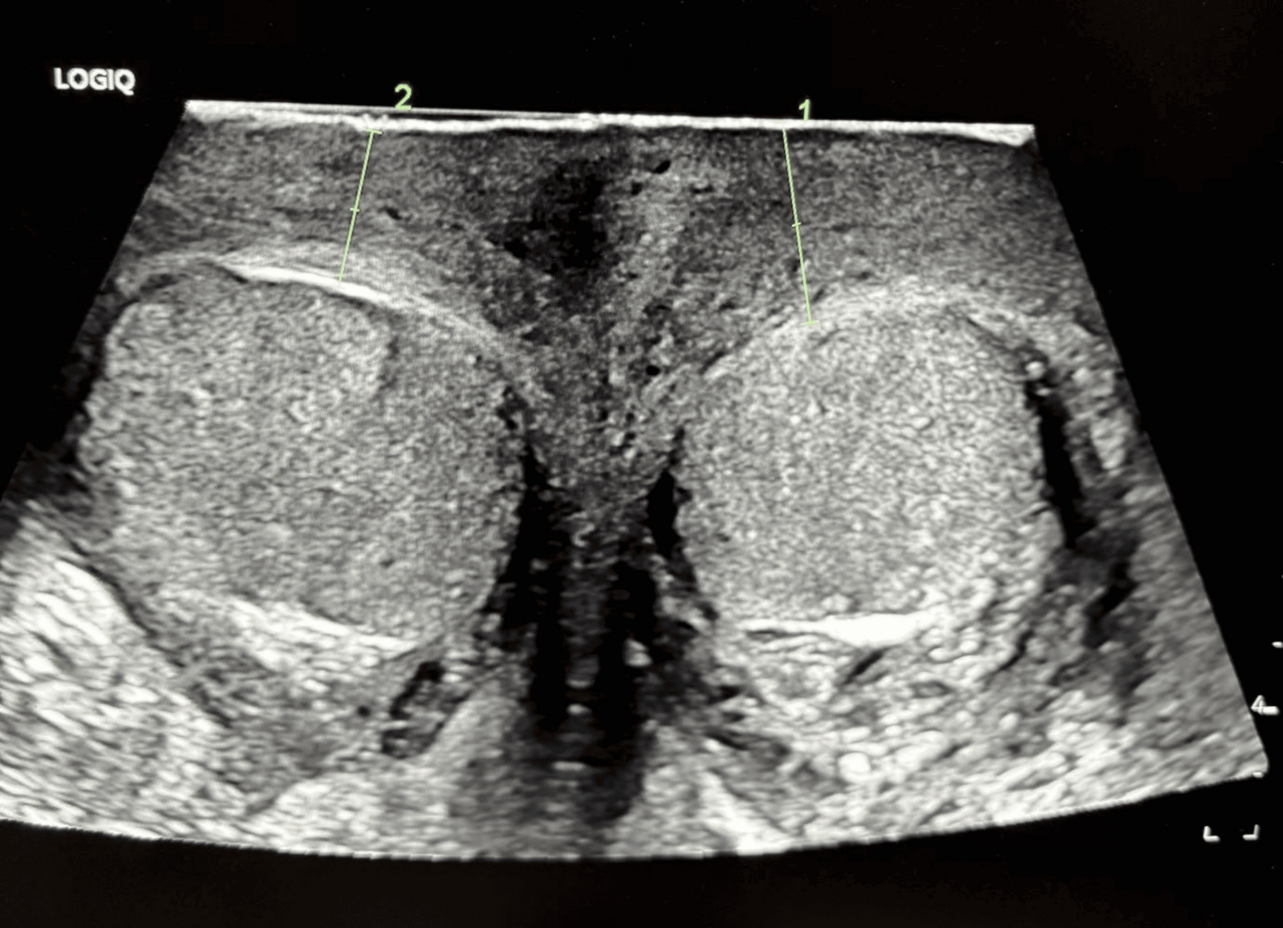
Ultrasonogram of scrotum: diffuse scrotal swelling without cutaneous abscess (area within 1 and 2).
